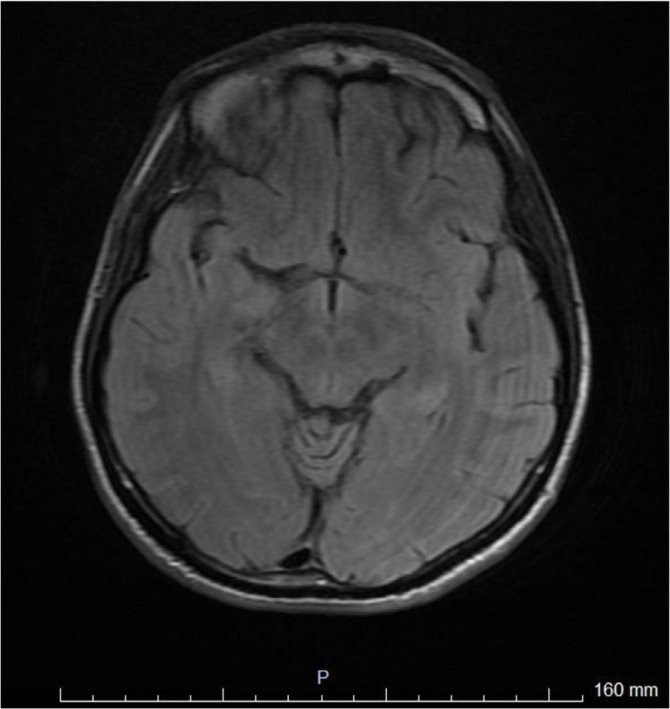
FLAIR MRI; complete resolution of vasogenic edema after supportive treatments
